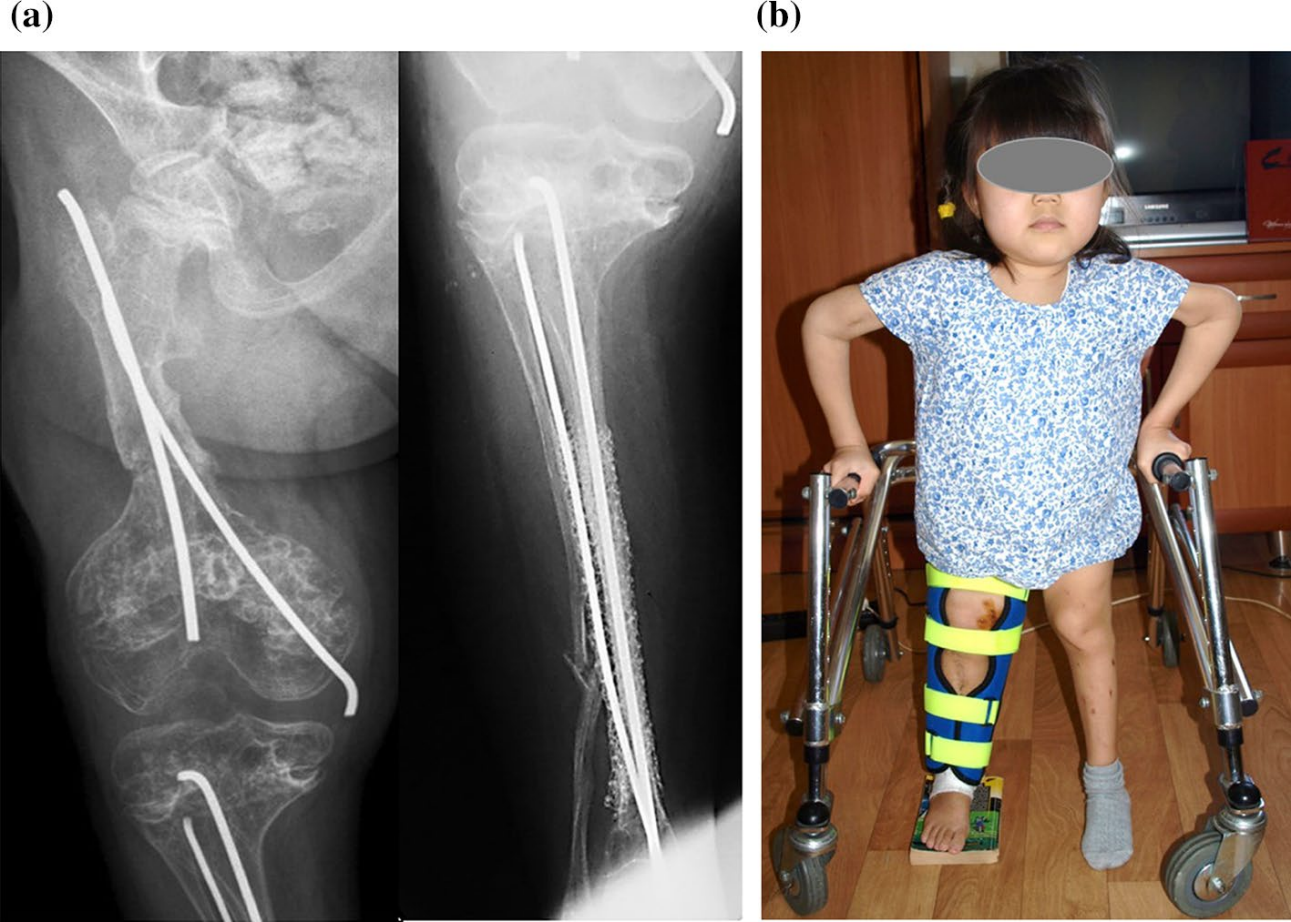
After fixator removal: a X-radiographs of right femur and tibia on the day of external fixator removal; b patient in 2 days after fixator removal
